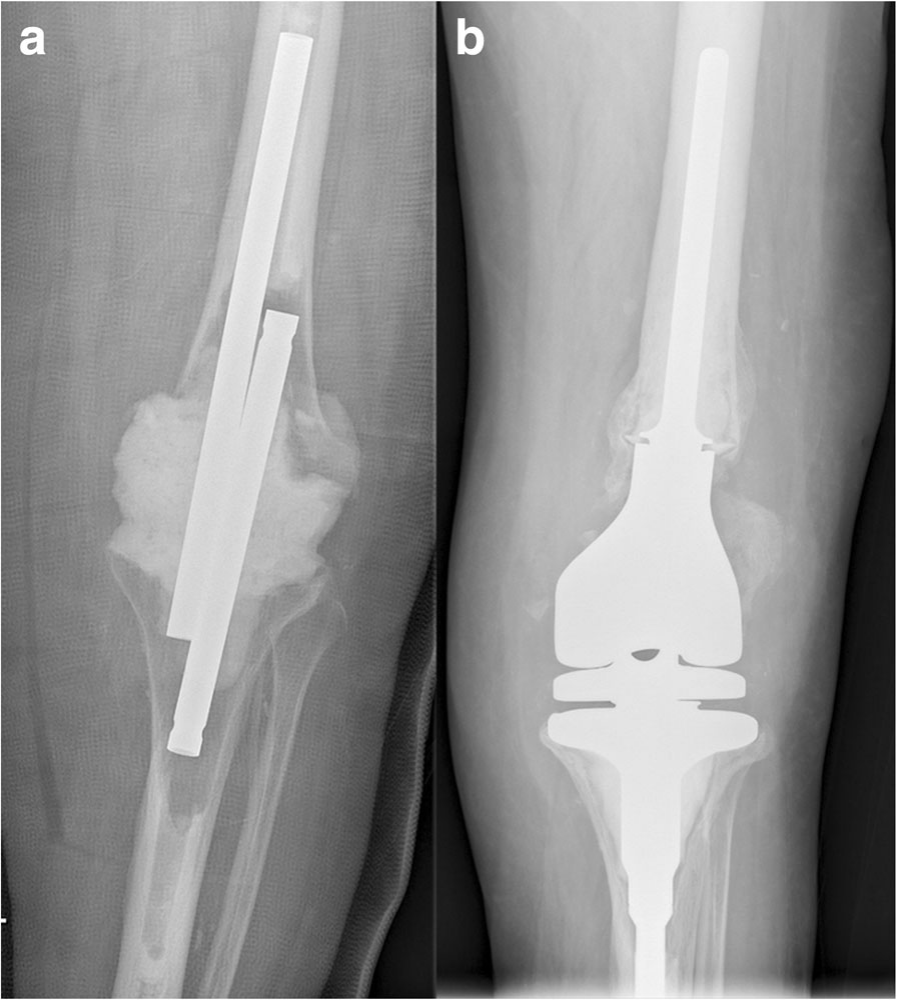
a Anteroposterior radiograph of a 72-year old female patient with arthrodesis and antibiotic bone cement spacer due to prosthetic joint infection after TKA; b Postoperative radiograph after DFR with a modular distal femoral GMRS
